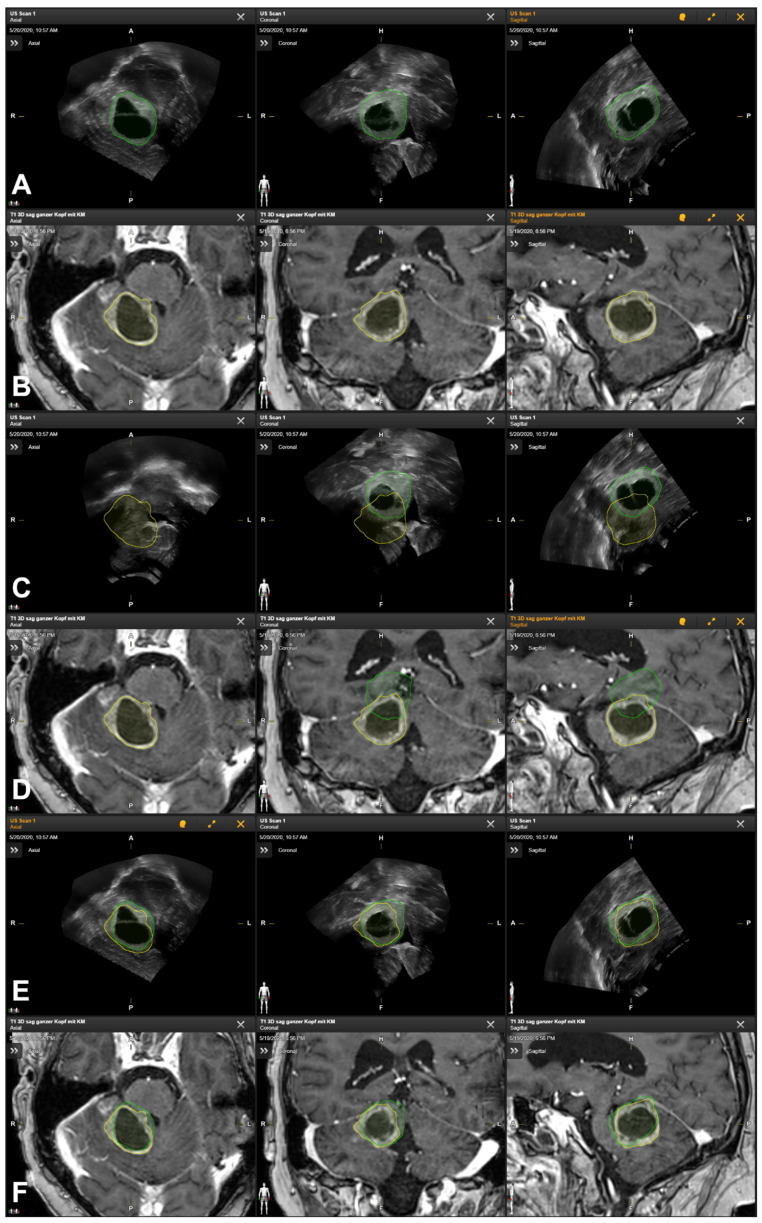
Manual segmentation of tumor outlines based on preoperative MRI data (segmentation in yellow, (A)) and navigated intraoperative US data (segmentation in green, (B)) showing the spatial mismatch of preoperative MRI-based tumor outlines and intraoperative iUS data leading to an iUS-based navigation update by manually outlining the tumor within the intraoperatively acquired US data set further used for navigation purposes in case of reference array-based registration (C,D) as well as good match between both data sets after rigid image-based registration of MRI and iUS data (E,F).
